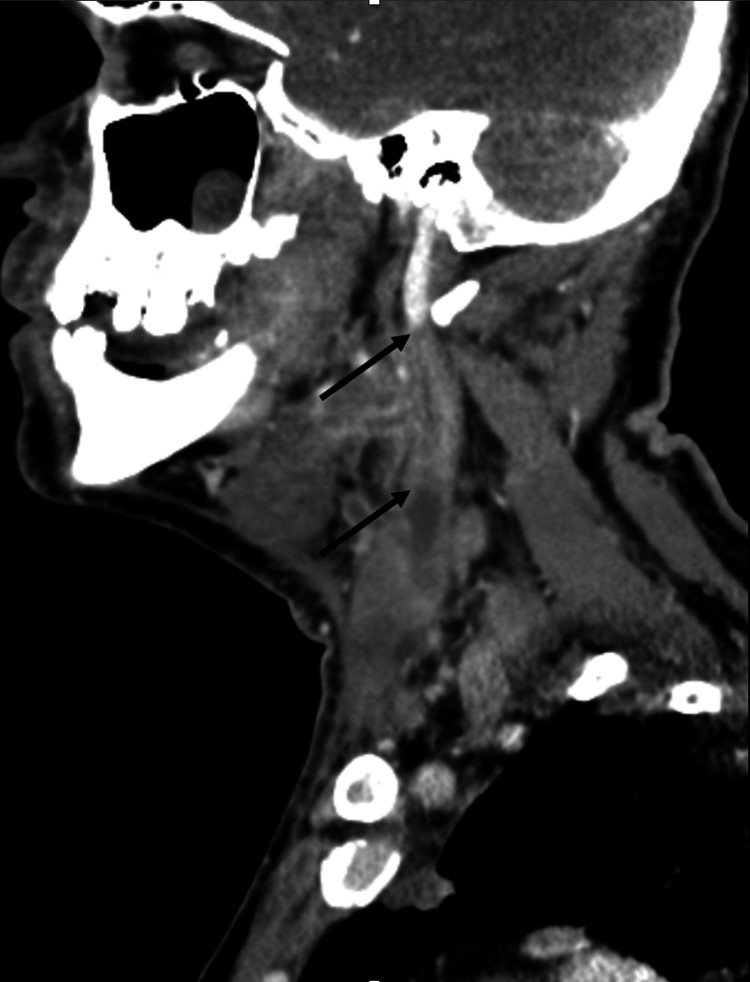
Repeat sagittal contrast-enhanced CT showing complete occlusion of left internal jugular vein indicated by the black arrows.
